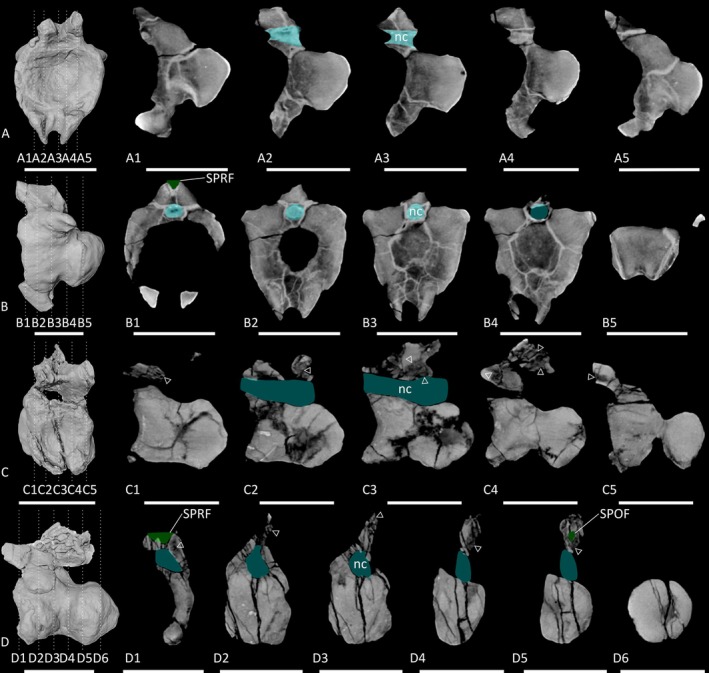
CT scan of indeterminate titanosaur caudal vertebrae. (A–D) 3D rendering (A, C) anterior view, (B, D) left lateral view; (A, B) Titanosauria indet. anterior caudal vertebra MAU‐Pv‐LI‐601, (C, D) Titanosauria indet. middle caudal vertebra MAU‐Pv‐LJ‐472/1; (A1–A5, C1–C5) CT scan slices in parasagittal cross‐section, (B1–B5, D1–D6) CT scan slices in transverse cross‐section. Nc, neural canal; SPRF, spinoprezygapophyseal fossa. Key: Blue highlight, neural canal; green highlight, neural spine fossa; white arrow, pneumatic bone texture. Scale bars = 100 mm.
